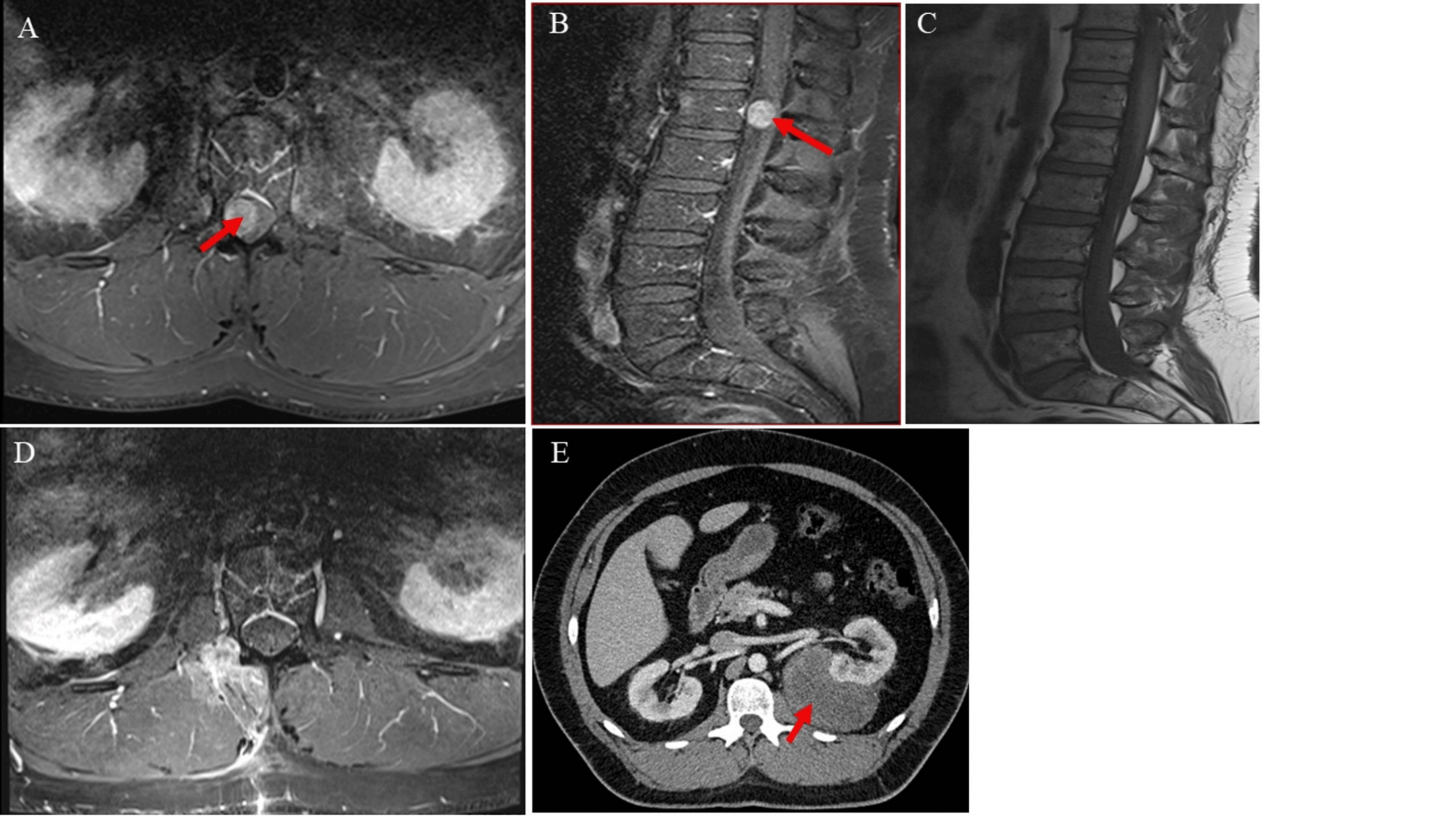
Images of the mass lesions: lumbar region (pre- and postoperative) and perirenal region. (A, B) Axial and sagittal T1-weighted MRI of the lumbar spine with contrast demonstrating a well-defined, homogeneously contrast-enhancing spinal mass at the L1/L2 level, measuring 3.5 cm transversely, 1.5 cm anteroposteriorly, and 2.5 cm cranio-caudally (red arrow).
